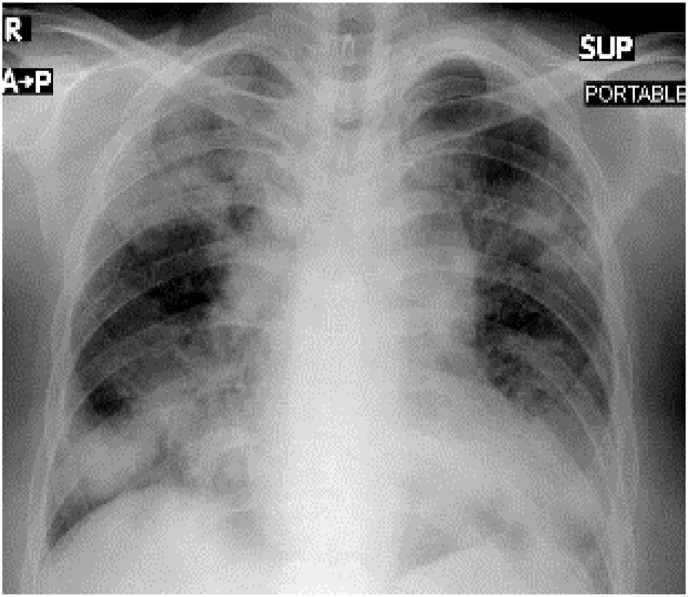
Chest x-ray, posteroanterior (PA) view, January 2019: Bilateral peri-hilar, peri-bronchial cuffing with diffuse, bilateral alveolar shadows more in the right lung.
